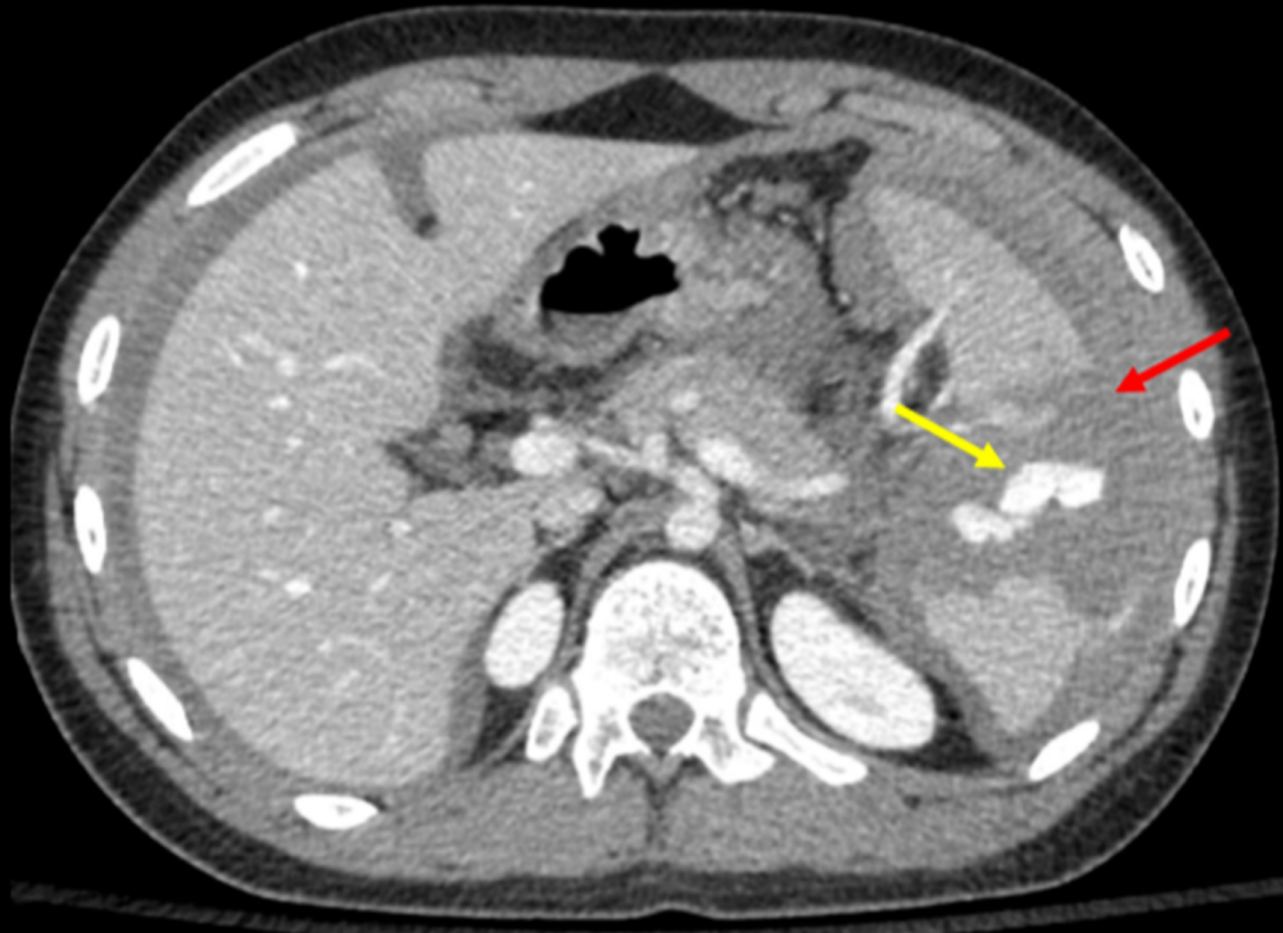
Initial Contrast-enhanced Computed Tomography of Splenic Injury The contrast-enhanced computed tomography of the patient's spleen shows a splenic injury, as a result of blunt force trauma. The yellow arrow shows active contrast extravasation, and the red arrow shows a hematoma.
